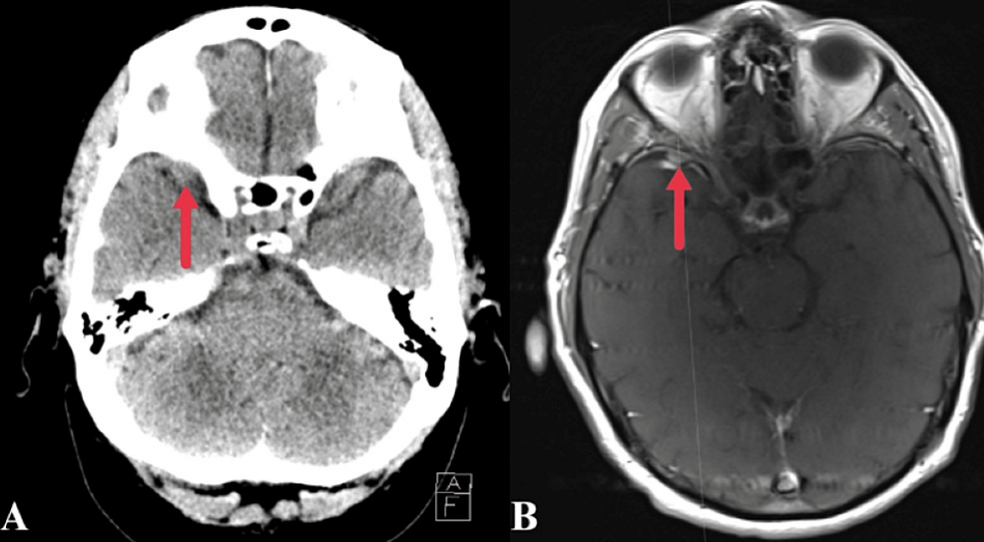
Axial CT (A) and T1 contrast-enhanced MRI (B) images performed two years prior demonstrate the same lesion, measuring 6 x 6 x 6 mm
